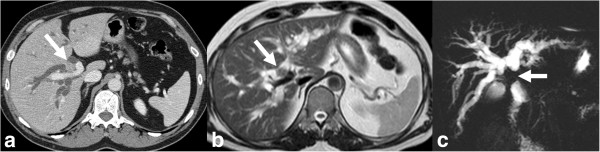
CCA of common hepatic duct with involvement of confluence. Contrast enhanced CT (a), T2-weighted MRI (b) and MRCP (c) images demonstrating thickened and enhancing common hepatic duct (arrow) with involvement of the confluence and upstream dilatation of the intrahepatic ducts. The ductal thickening appears hypointense (arrow) to the surrounding dilated bile ducts on T2-weighted MRI image (b). The involvement of the confluence is demonstrated better on MRCP.
